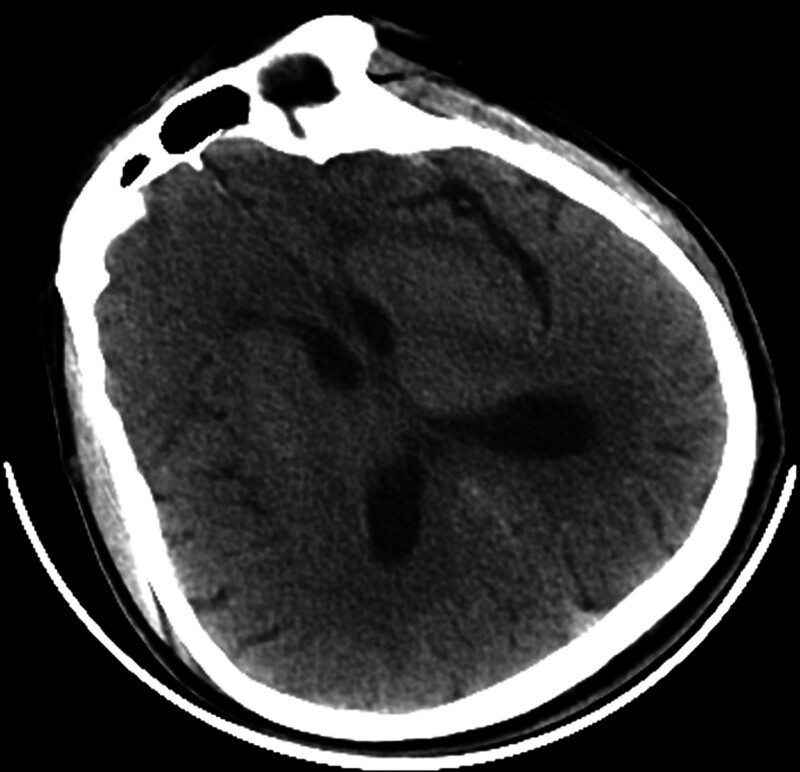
A CT scan of the head on the fifth day of illness still showed no abnormal findings. CT = computed tomography.
